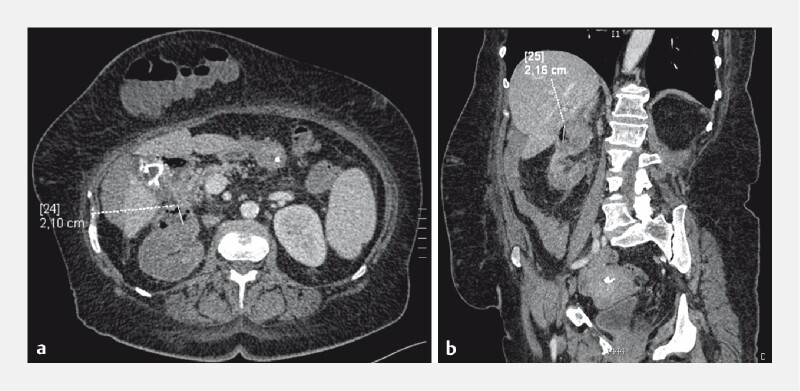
Computed tomography performed 5 months after the procedure: successful endoscopic treatment (collection size 21 × 10 mm; decrement > 50 %).
